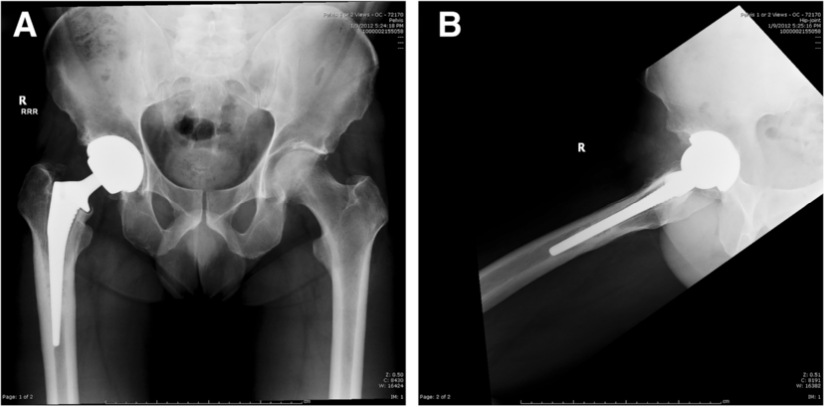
Pre-operative anteroposterior (AP) and lateral hip radiographs (A-B) demonstrating metaphyseal loosening of the femoral component.
